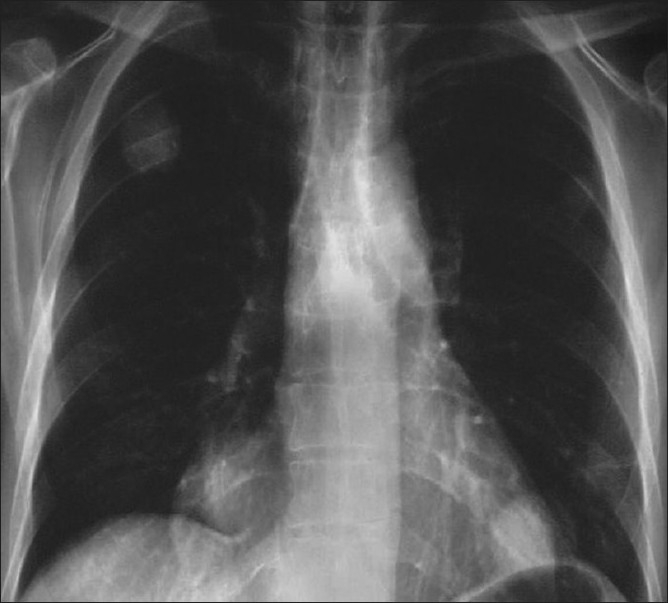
Lymphoma. Chest X-ray (CXR) on a human immunodeficiency virus patient that presented with multiple lung masses, which grew rapidly mimicking infection. Note that there is no associated lymphadenopathy. Well-defined solitary or multiple parenchymal nodules CXR are common. A percutaneous biopsy revealed a non-Hodgkin’s lymphoma
